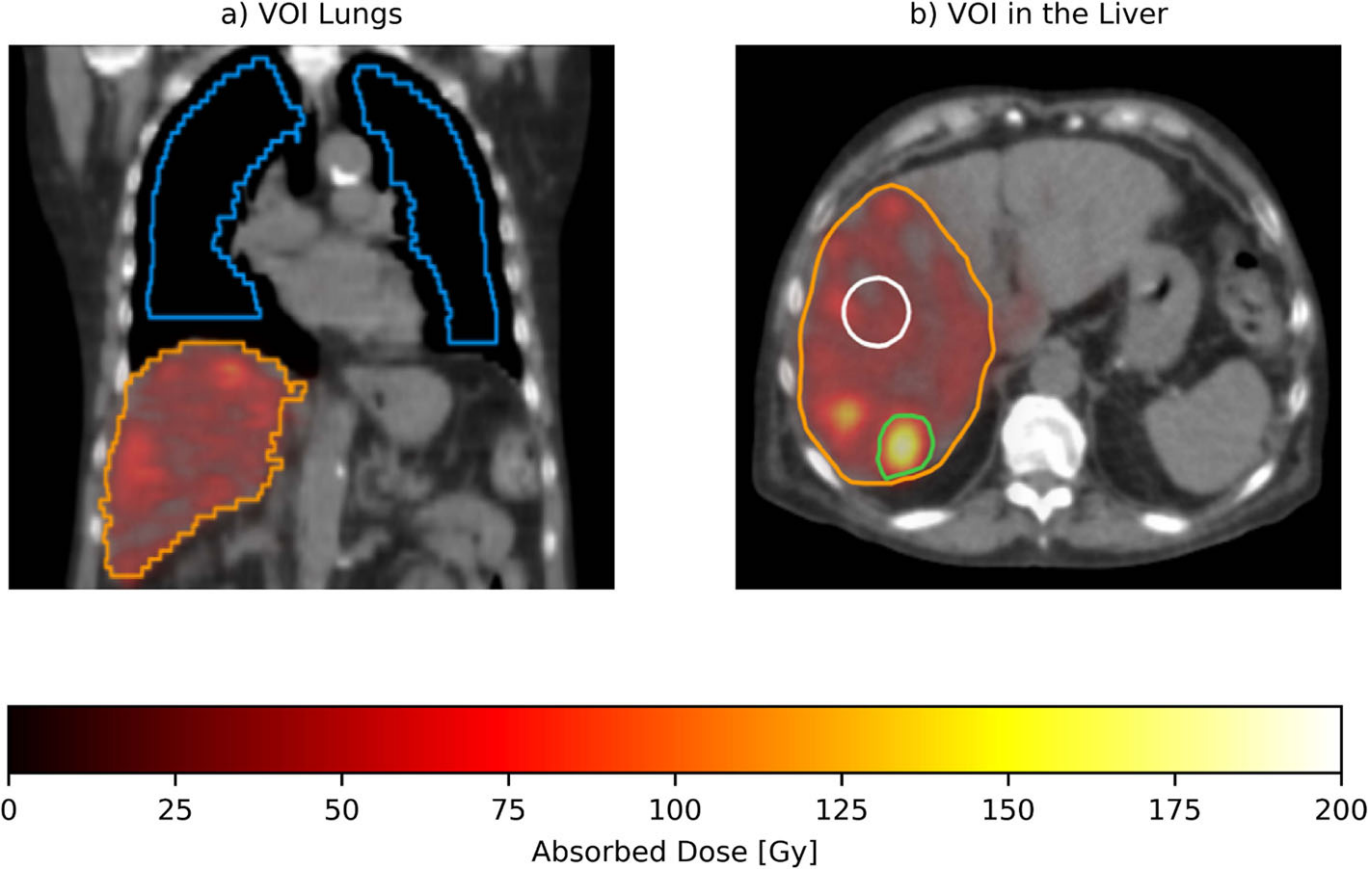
^166^Ho-SPECT/CT images of a 70-year-old male diagnosed with multiple liver metastases of neuroendocrine origin after receiving a ^166^Ho radioembolization treatment (right liver lobe, 4207 MBq). a Coronal view where the orange line defines the treated liver, and the blue line depicts the semi-automatically delineated lungs, after the shrinkage process. b Axial view of the liver with 3 VOIs superimposed. Orange line delineates the treated liver (right lobe), and green line delineates the tumor (VOI_TUMOR_) while white line defines the VOI_HEALTHY_
